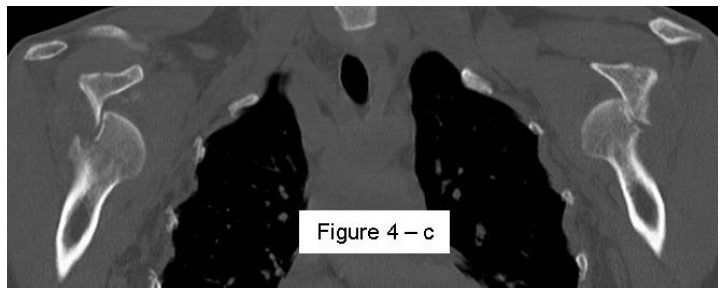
CT coronal view of the same case 111x44mm (150 x 150 DPI).
